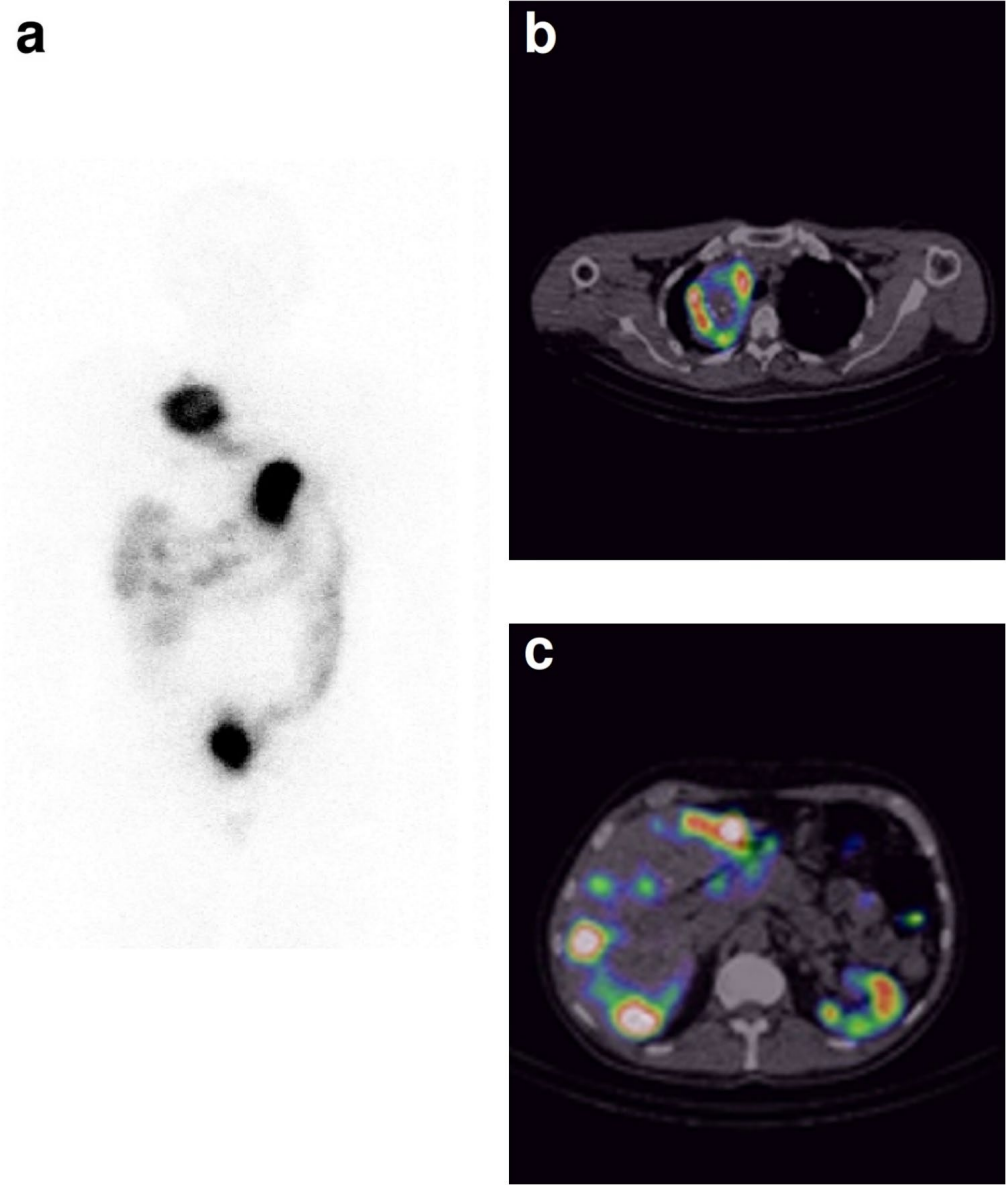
A 49-year old male (MTC10) with advanced sporadic MTC, basal Ct 1288 pg/mL. Uptake of [^111^In]In-CP04 in a large tumour mass in the mediastinum on the right (b) and numerous liver metastases (c) is visible with considerable detail on SPECT/CT in comparison to planar (a) imaging
